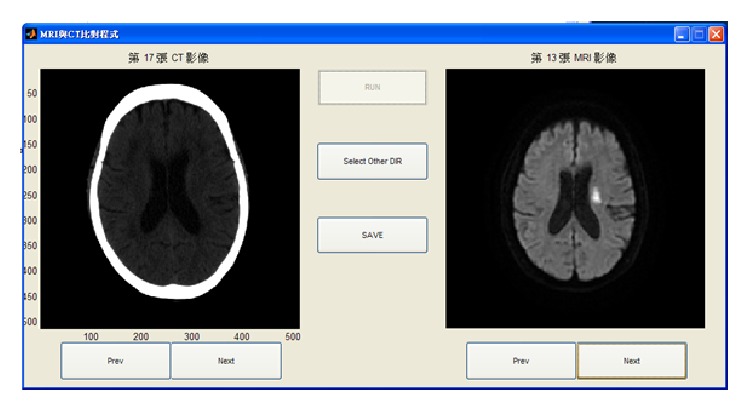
Typical user interface for obtaining an assessment by a professional radiologist. The left side shows a stroke patient CT image and the right side shows the corresponding MRI image of the same patient.
